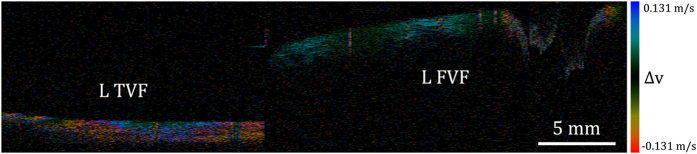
Doppler image of a B-frame. The subject was phonating at about 250 Hz. The image shows both left true and false vocal folds. The velocity distribution patterns are seen in both folds. However, the magnitude in the false vocal fold is much less than the true vocal fold. L TVF = left true vocal fold; L FVF = left false vocal fold.
